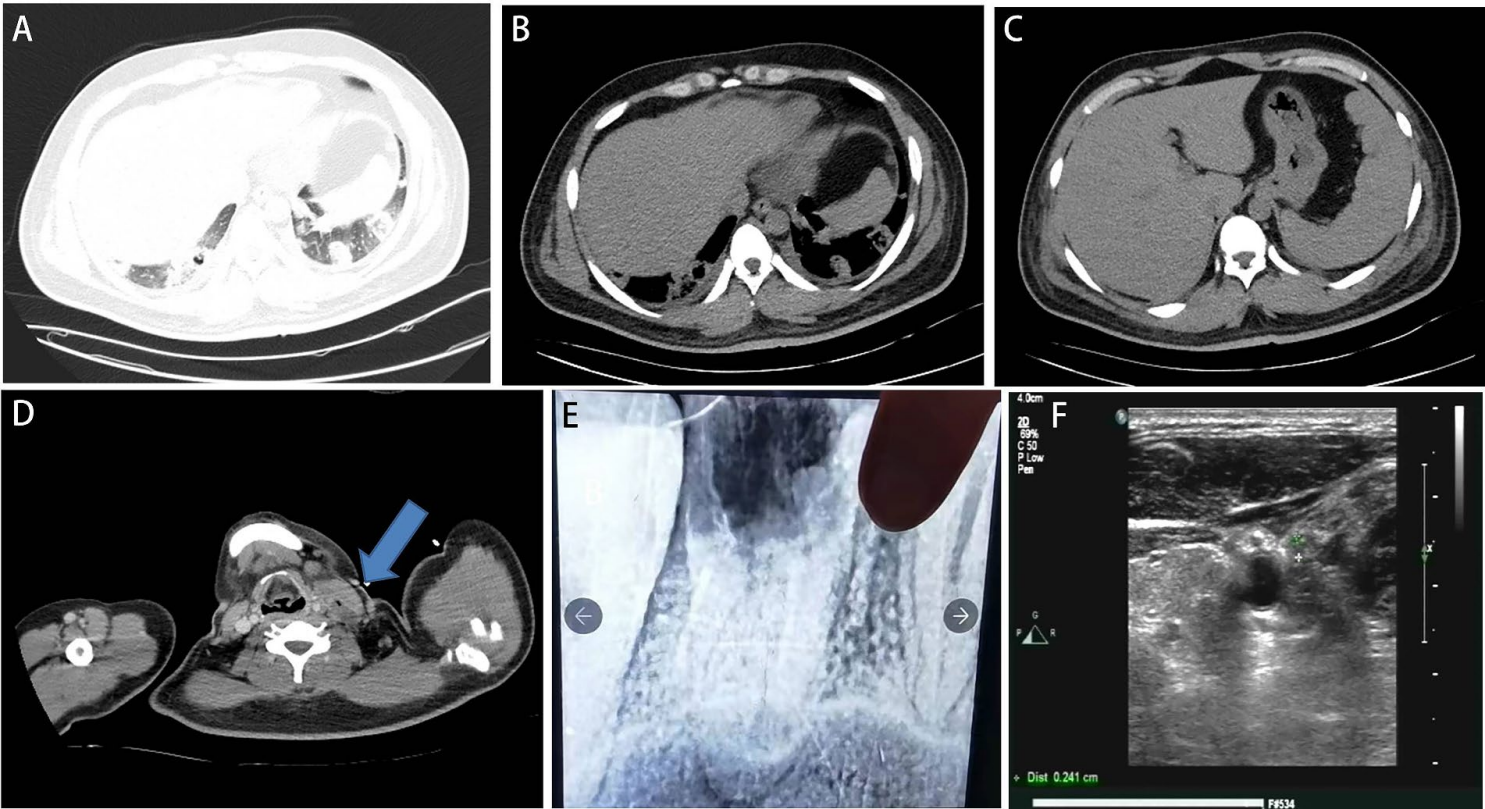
(A–D) Computed tomography scan at admission to the intensive care unit. (A,B) It showed bilateral pulmonary nodules and cavities. (C) Decreased liver density. (D) Left internal jugular vein thrombosis with gas accumulation (blue arrow). (E) X-ray after charge in a dental clinic. A cavity was at the left maxillary first premolar. (F) 2 months after discharge, the ultrasound showed that the thrombus in the neck had not subsided.
